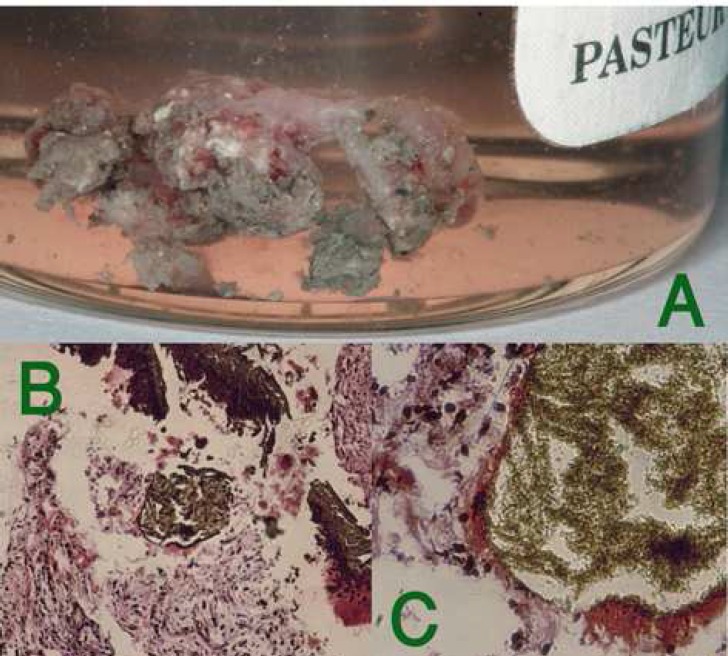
Mass of unset MTA extruded attached to the soft tissue removed from periapical tissue; B) MTA surrounded by granulation tissue, macrophage and fibroblasts; C) MTA particles encapsulated in the fibrous connective tissue
